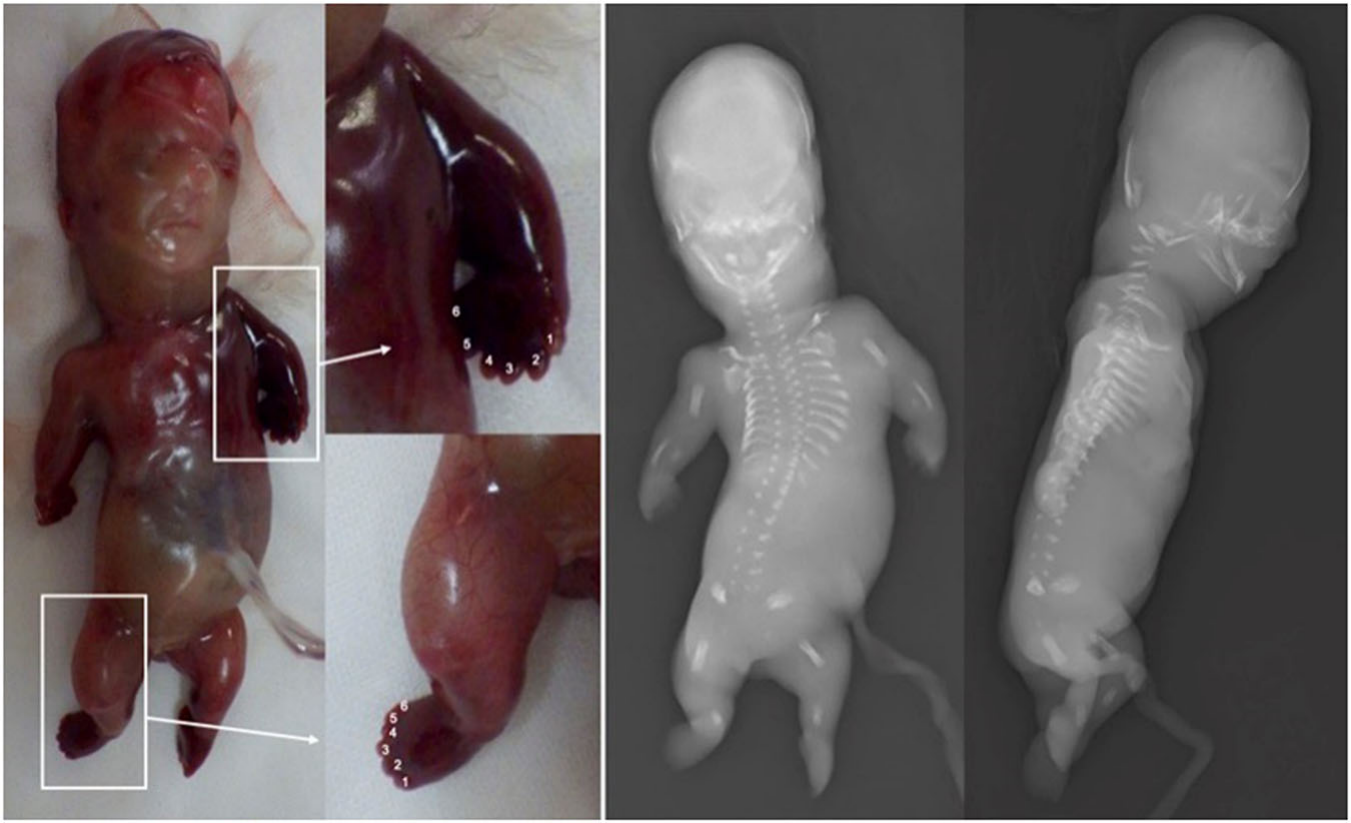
Left: The terminated baby presented an extremely small thoracic cage and short arms and legs, especially distal. Postaxial polydactyly and brachydactyly in the hands and feet are denoted with arrows. Right: Postmortem radiographs of the fetus showing generalized skeletal alterations with striking immaturity of the skeleton, including a severely narrow thorax with short ribs, defective ossification of the vertebral bodies and ilia, and absent ossification of the pubic and ischial bones. The long bones are severely short. The ulnae are particularly short, and the fibulae are not ossified. Ossification of the short tubular bones is absent.
